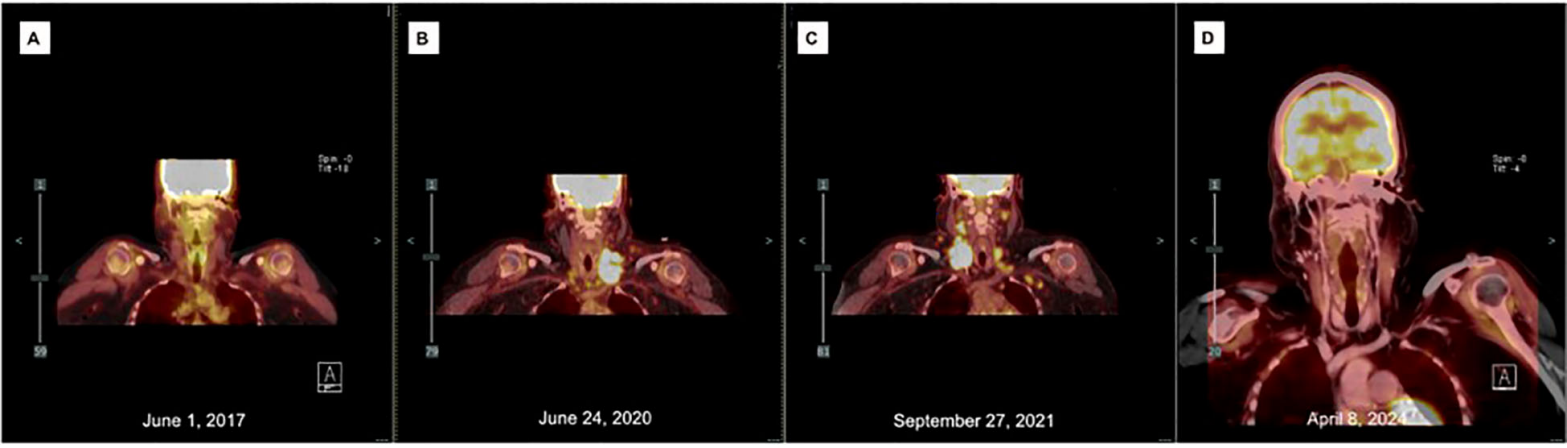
PET scans of the neck throughout treatment course: (A) At diagnosis, pathology consistent with Langerhans Histiocytosis, left supraclavicular LN measuring 1.3cm with SUV 2.2; (B) Transformation to Langerhans Sarcoma, left supraclavicular nodal mass measuring 4.5cm x 2.7cm with SUV 18.6; (C) Following six cycles of cladribine and seven cycles Pembrolizumab, right supraclavicular nodal mass measures 3.6cm x 2.8cm with SUV 16.1; (D) 12 months following allogeneic matched unrelated donor transplant, most prominent LN measures 6mm with SUV 2.3.
